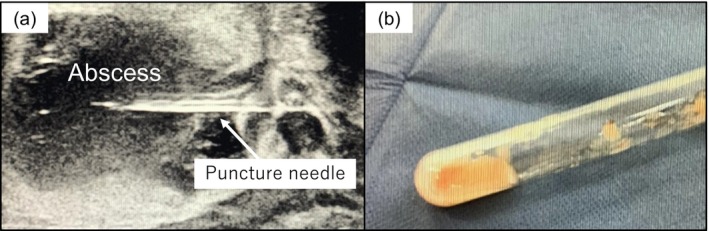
Abscess aspiration. (a) Ultrasound‐guided transperineal abscess drainage. (b) Pus retrieved from the abscess aspiration; the sample was highly viscous, and only a small volume was obtained.
